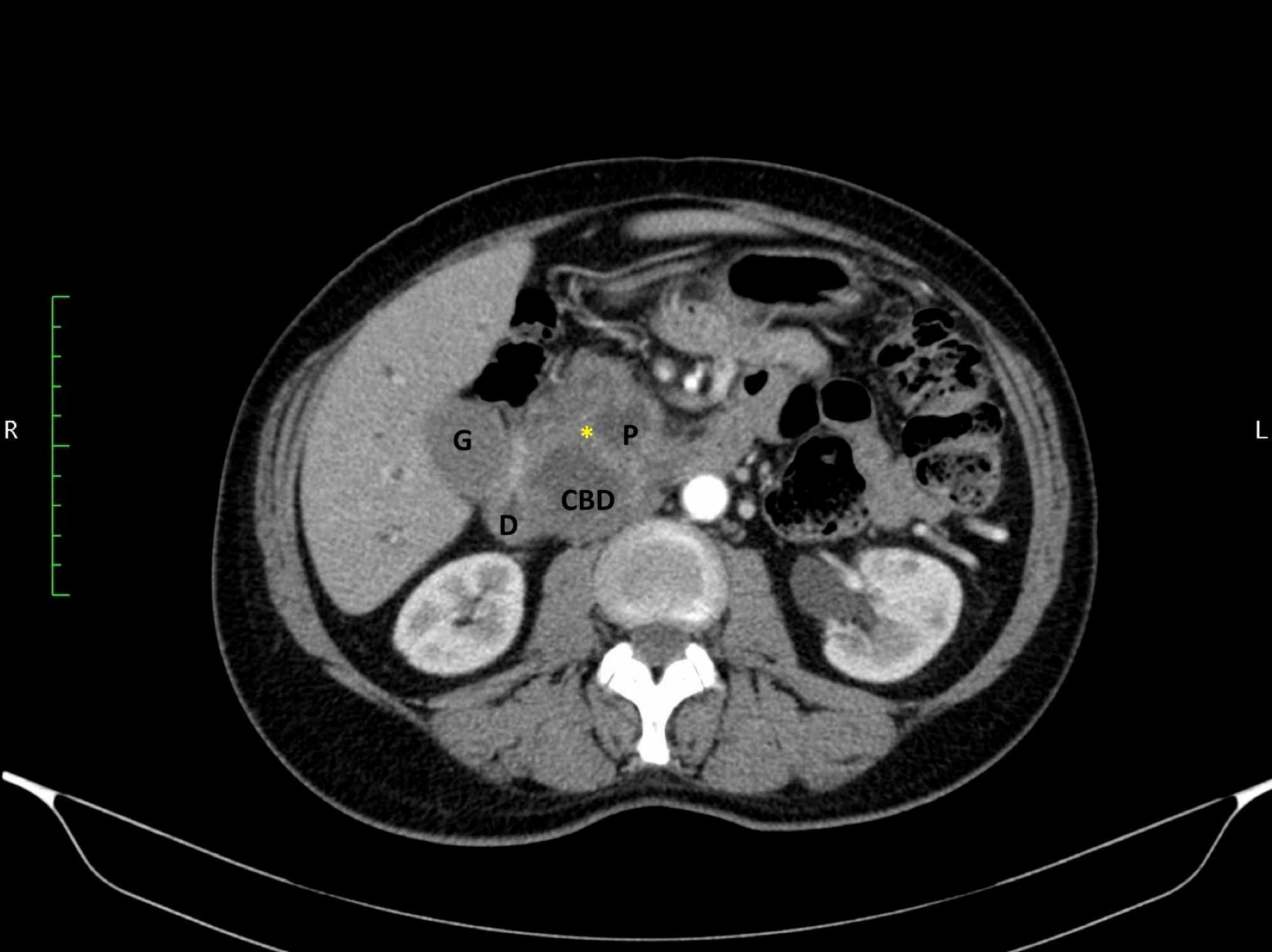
Axial CT scan images demonstrate a heterogeneous mass in the pancreatic head (asterisk) The common bile duct (CBD) and pancreatic duct (P) are also visible, forming a double duct sign. The dilated gallbladder (G) and duodenum (D) are also seen.
